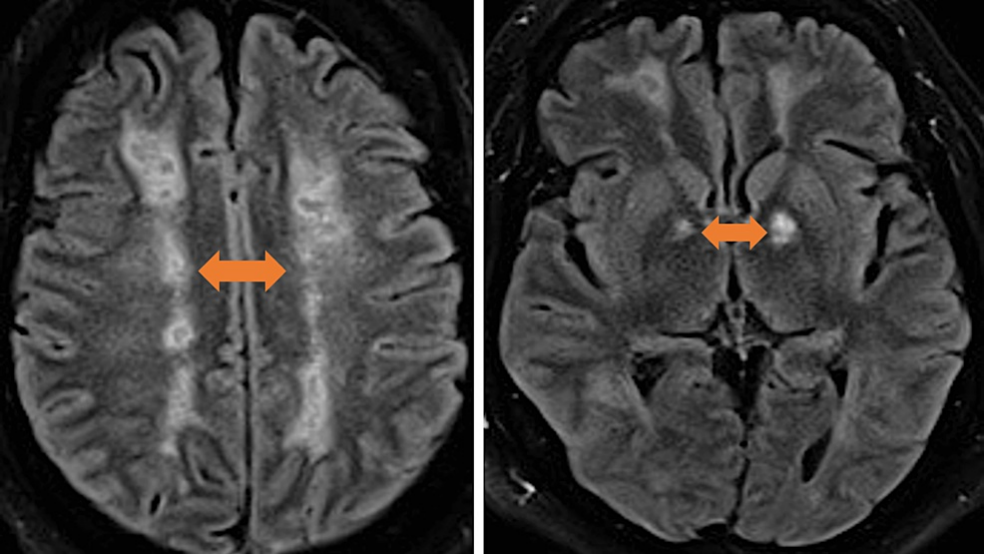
MRI of the brain T2/FLAIR sequence axial images with confluent white matter signal abnormality in bilateral frontoparietal and occipital white matter. Multiple small areas of cystic encephalomalacia in bilateral frontal and parietal deep white matter and bilateral globus pallidus (arrows). MRI: magnetic resonance imaging; FLAIR: fluid-attenuated inversion recovery
